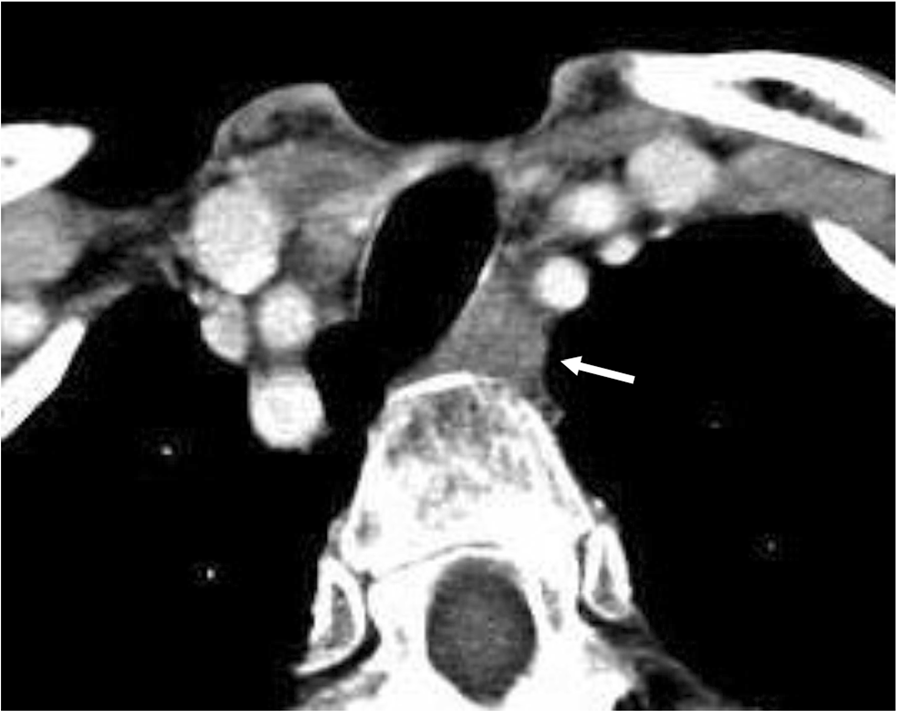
Computed tomography indicating recurrence in the paratracheal lymph node. The paratracheal lymph node is indicated with an arrow
